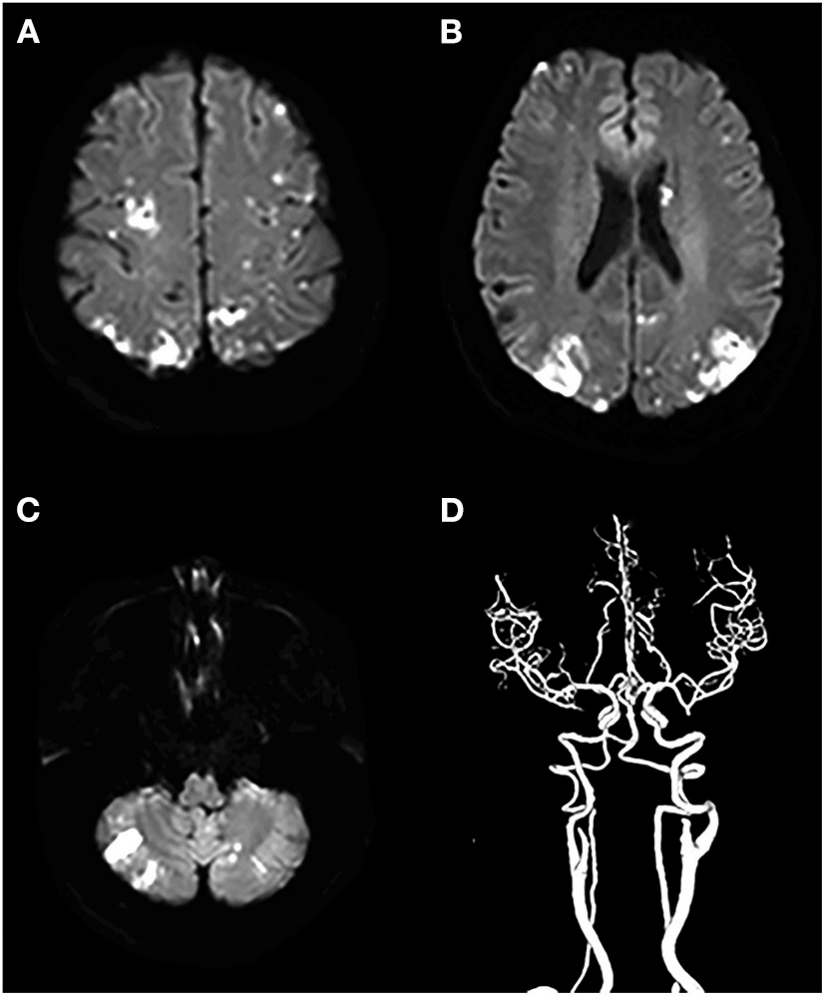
A cryptogenic stroke involving three circulations in a 72-year-old woman with a malignant tumor of the abdomen. The patient had no traditional risk factors for ischemic stroke such as atrial fibrillation, hypertension, diabetes mellitus, etc. (A–C) DWI images show multiple acute lesions in multiple vascular territories (in the bilateral anterior and the posterior circulations), and (D) shows no meaningful stenosis on CTA.
